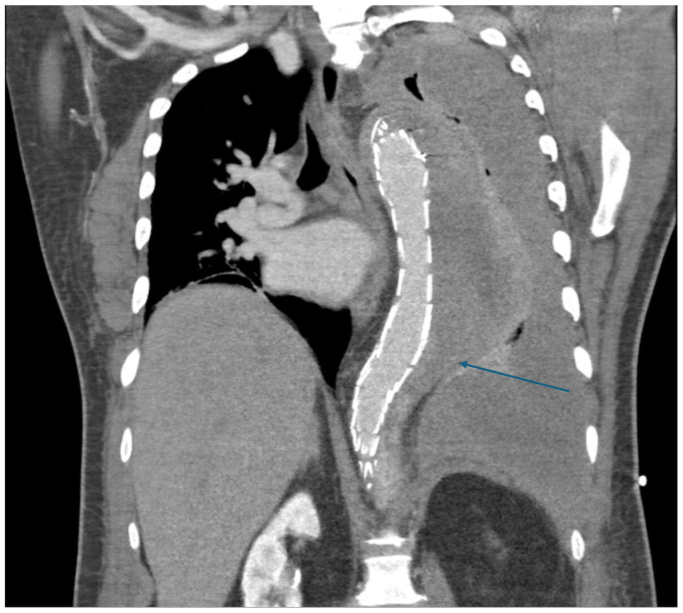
Computed tomography angiography (CTA) following thoracic endovascular aortic repair (TEVAR). A coronal image demonstrating endovascular graft in situ post repair with persistent retrograde flow within the false lumen (FL) and a large left-sided hemothorax. The *blue arrow* indicates the tapering segment of the FL, which was targeted for deployment of the occlusion device.
